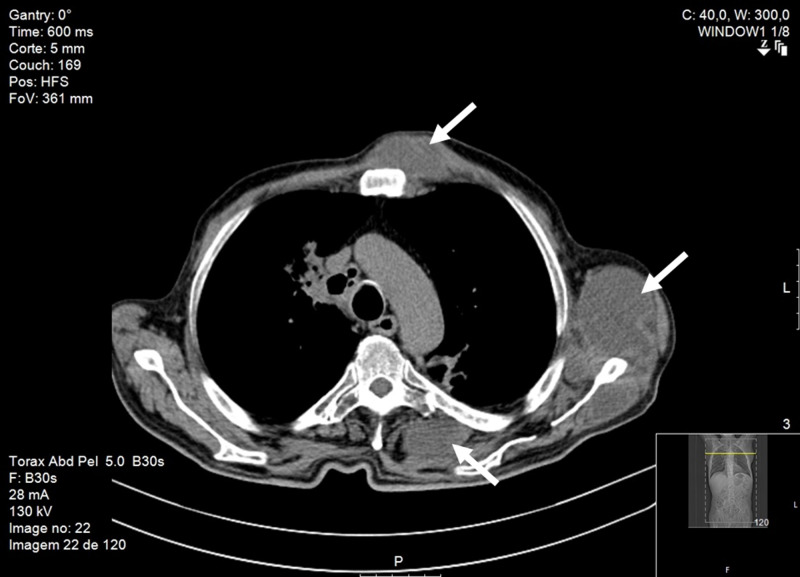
CT scan revealing parasternal, axillary and dorsal masses
